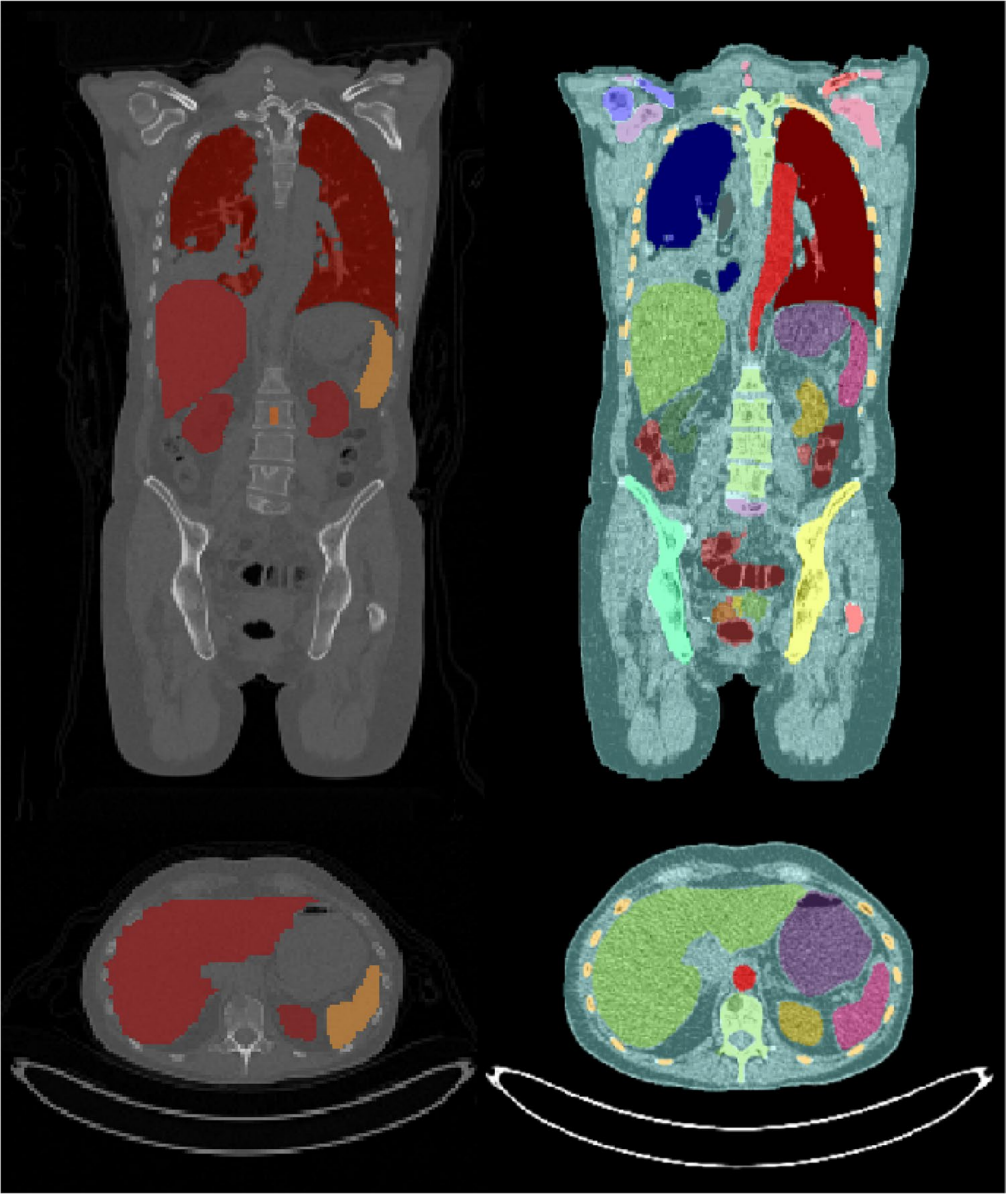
Low-dose CT example patient images in coronal view (upper row) and axial view (lower row) including whole organ segmentations performed manually (left column) and using the AI-based tool (right column)
